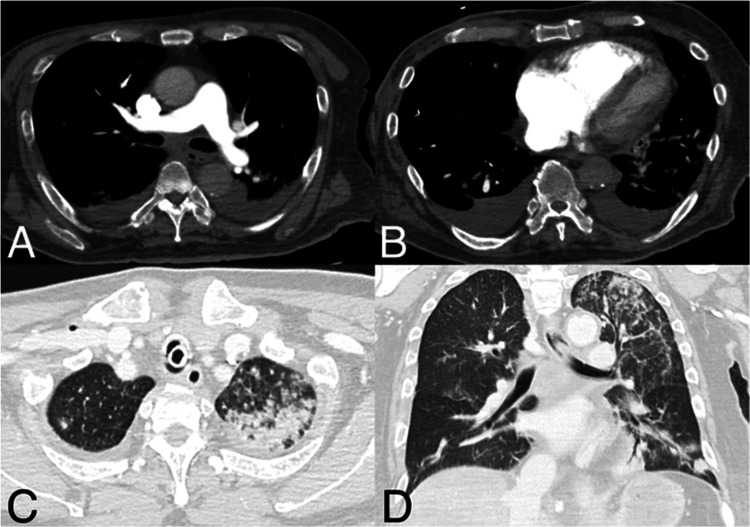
Contrast-enhanced computed tomography on the third day of hospitalization Contrast-enhanced computed tomography findings. (A) No pulmonary thromboembolism is observed in the pulmonary arteries. (B) Right ventricular enlargement, contrast reflux into the inferior vena cava, and bilateral pleural effusions are present. Periportal collar signs and subserosal edema of the gallbladder (not shown) are noted, suggesting acute right heart failure secondary to right ventricular overload. (C, D) Centrilobular nodular opacities, consolidation, and interlobular septal thickening were observed in the left upper lobe, and inflammatory changes and carcinomatous lymphangitis were considered in the differential diagnosis.
